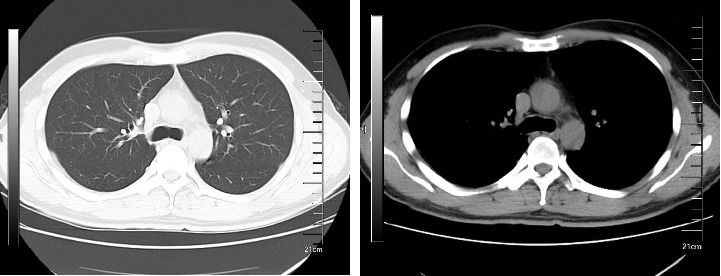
Bilateral lung nodules as indicated by the chest CT, and recommended follow-up for Case 1.
